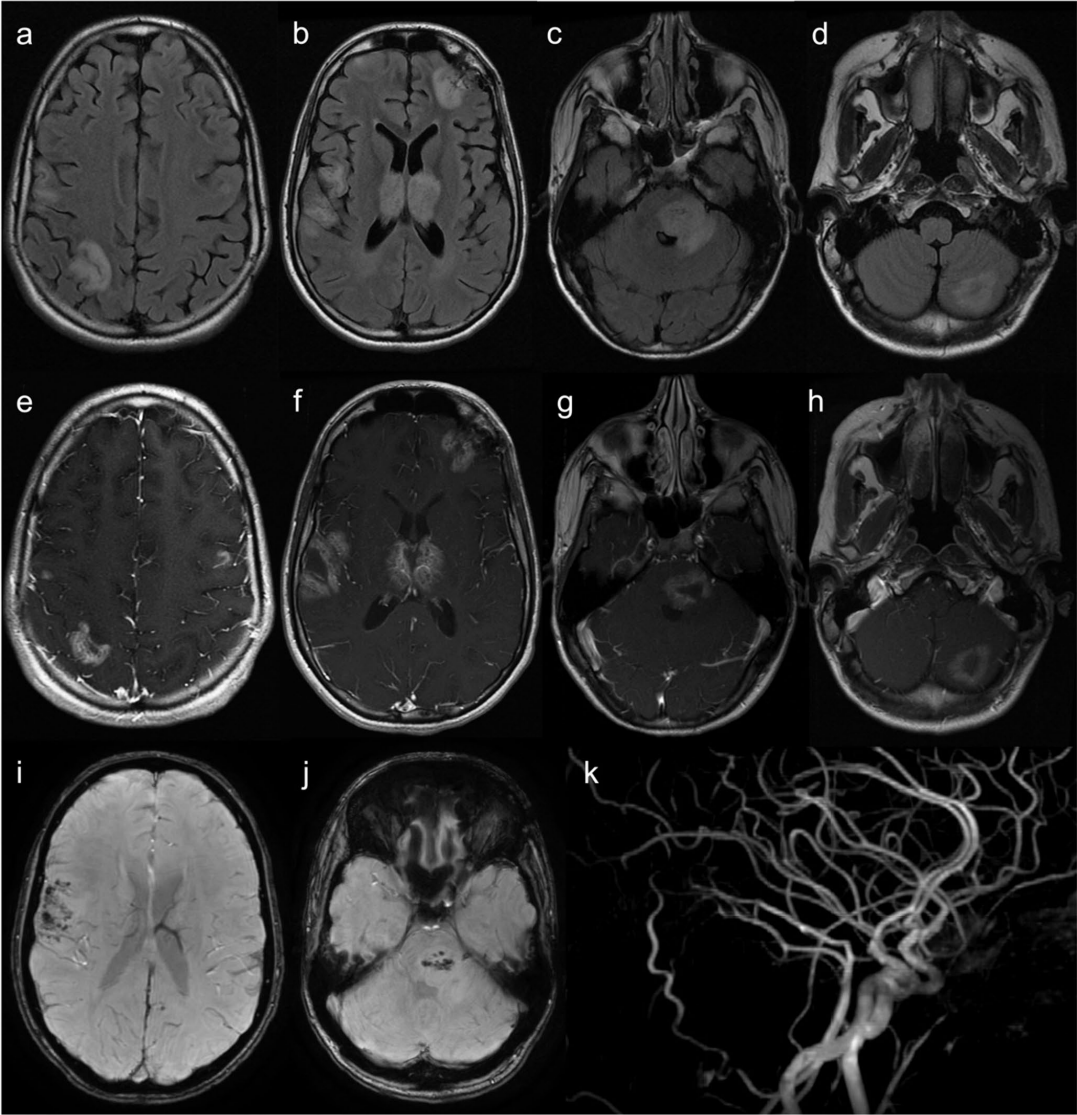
X-linked lymphoproliferative disease type 1. A 16-year-old male with behavioral changes and a history of intestinal Burkitt lymphoma resected two years earlier. Brain MRI (a to d – axial FLAIR, e to h – gadolinium-enhanced axial T1, and i and j – axial SWAN) shows multifocal edematous supra and infratentorial lesions, predominantly affecting the cortico-subcortical regions, with heterogeneous enhancement and some hemorrhagic foci. (k) No abnormalities were detected in the 3D reconstruction of the TOF MR angiography. Despite the bithalamic involvement with hemorrhage, also seen in parainfectious ANE (Fig. 11), the progressive clinical course (not depicted), presence of multiple asymmetric cortico-subcortical lesions, intense enhancement, and sparing of the internal and external capsules, and medial temporal regions are, in this case, more consistent with CNS vasculitis. This diagnosis was confirmed by brain biopsy, which excluded lymphoma
